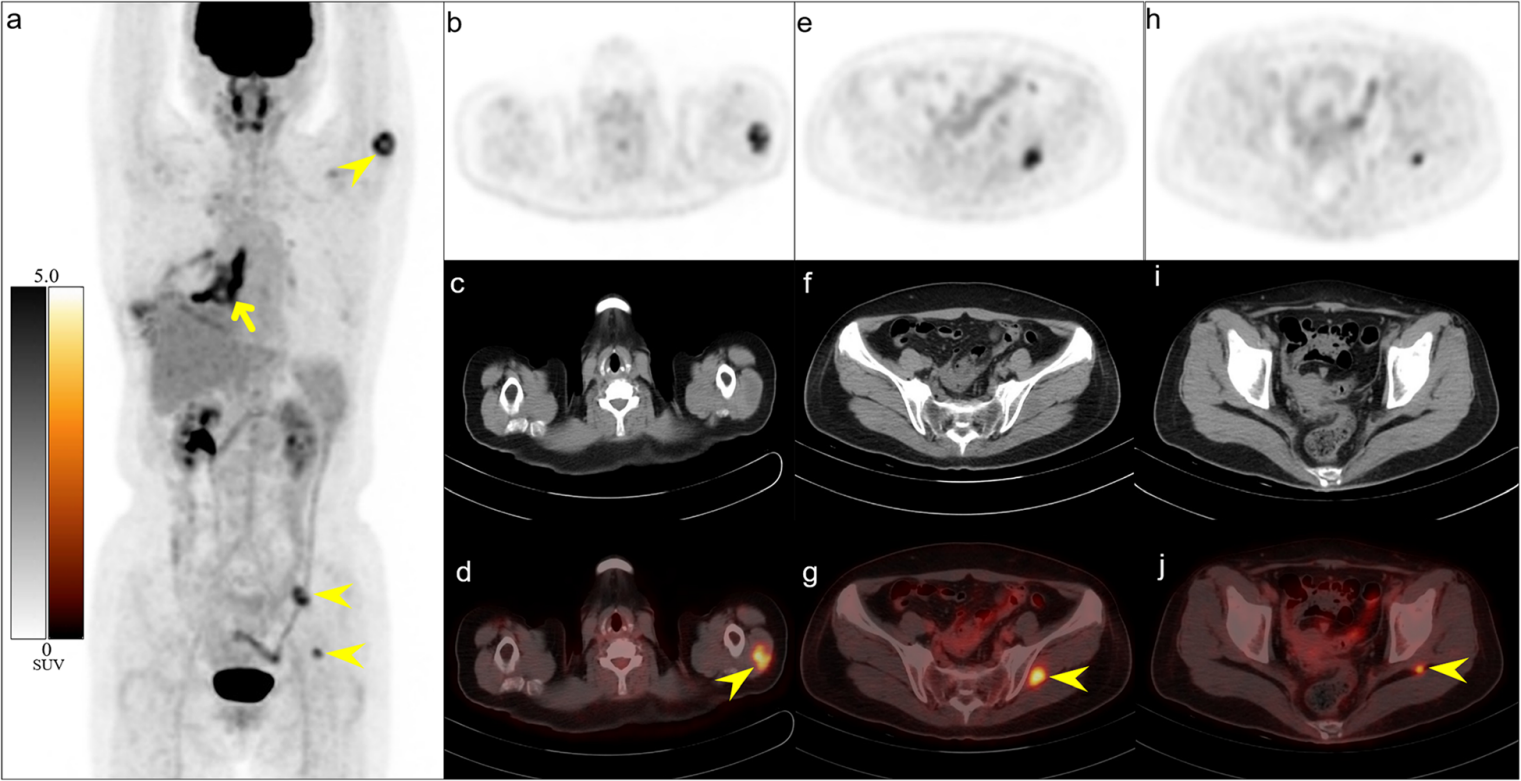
STM changed the postoperative stage of a lung squamous cell carcinoma. A 62-year-old woman was referred to our hospital with a 2-month history of cough. Squamous cell carcinoma of the lower lobe of the right lung was diagnosed by chest CT and lung biopsy. The general condition of the patient was good, and no metastases were found in head MRI or thoracic and abdominal CT. The patient underwent surgical resection and received adjuvant chemotherapy after the operation. Three months later, the patient underwent ^18^F-FDG PET/CT to assess treatment efficacy. MIP (a) showed increased ^18^F-FDG uptake (SUVmax = 3.8) in the operative area of the right lung (short arrows). MIP (a), axial images of neck and pelvis (e-j) revealed localized reduced-density nodules in the left deltoid muscle, left gluteus medius muscle, and left gluteal muscle (arrowheads), with FDG uptake (SUVmax = 8.0). Therefore, a diagnosis of multiple STM after lung cancer resection was considered. The patient was treated with palliative radiotherapy and chemotherapy to control the disease
